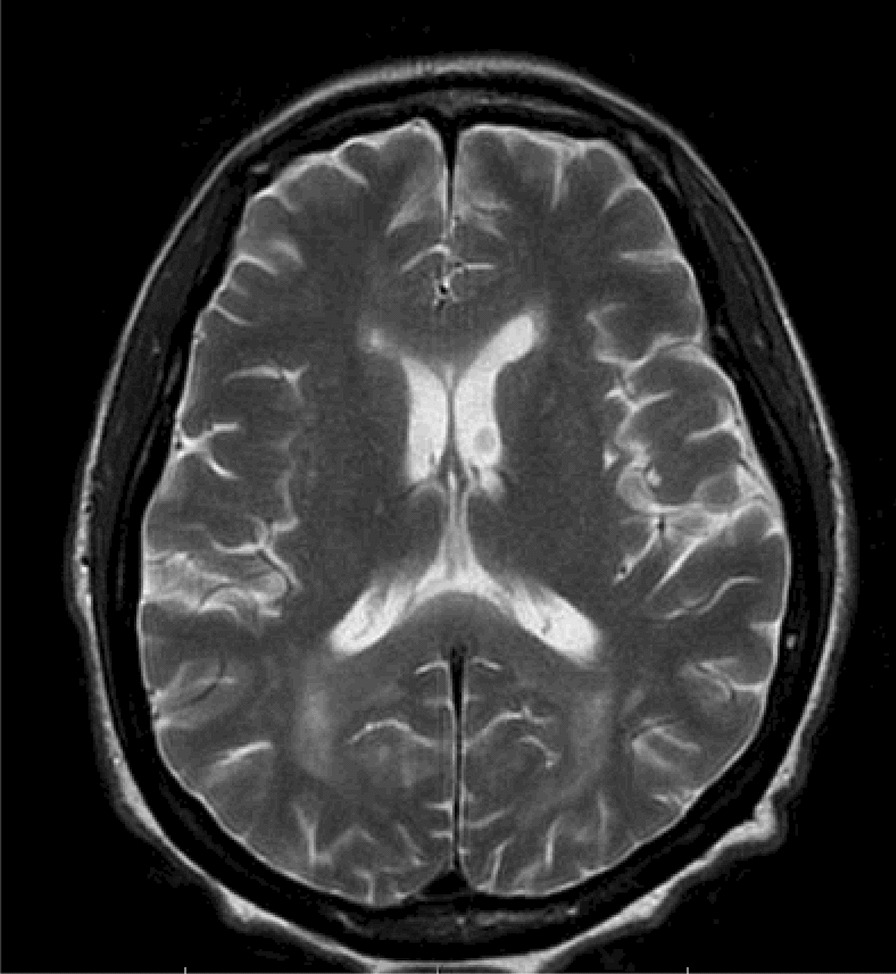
MRI brain with contrast showing significant confluent hyper signal T2 FLAIR within the subcortical and deep ventricular white matter of both cerebral hemispheres, as well as in the corpus callosum, right posterior limb of the internal capsule and cerebral peduncle
